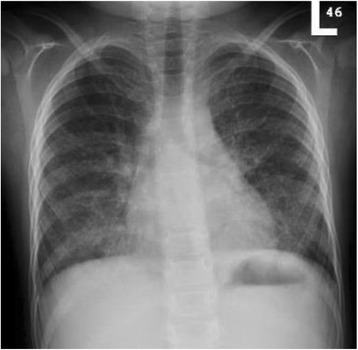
Chest X-ray 11 months after whole lung lavage with marked radiological improvement
